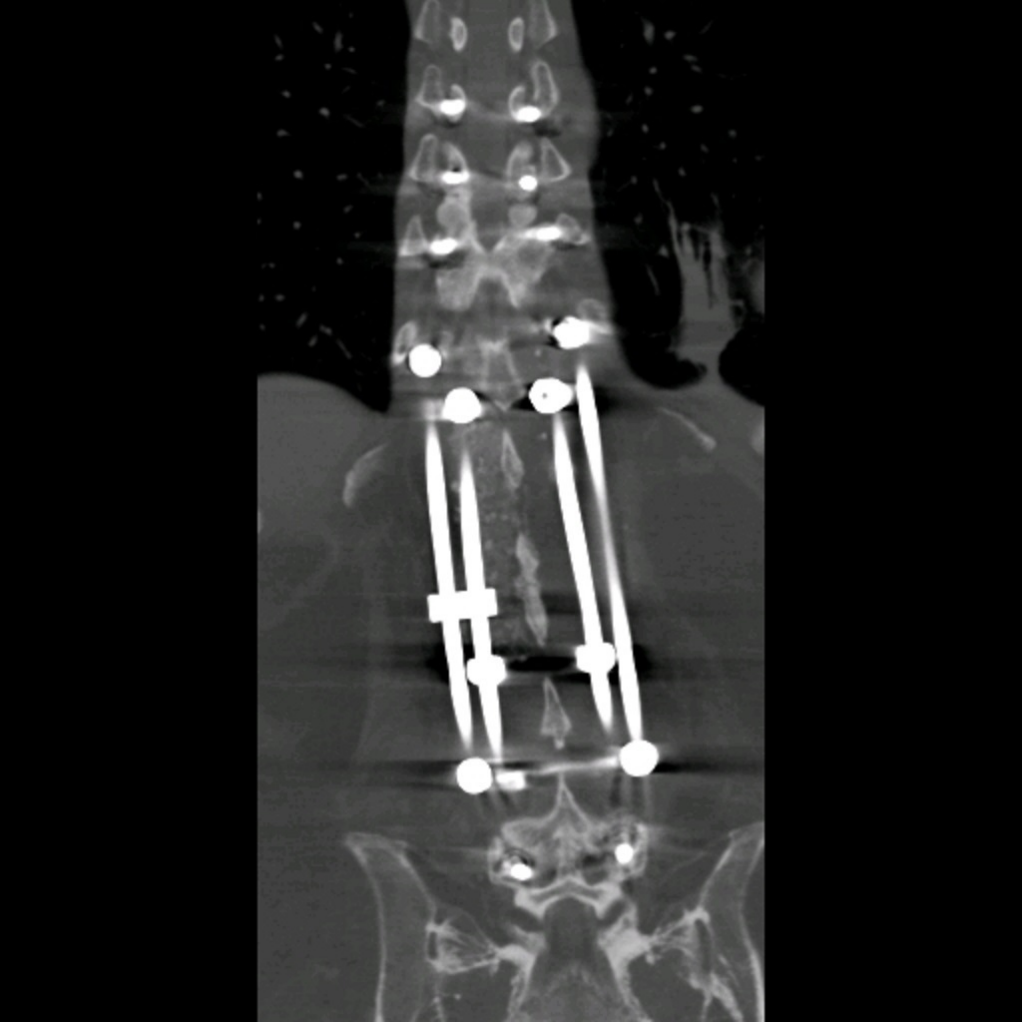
Coronal postoperative CT This coronal view of the postoperative CT of the thoracolumbar spine demonstrates the four-rod construct that was utilized. Medial and lateral entry points for pedicle screw placement were alternated from T8-12 and L3-5, as shown, prior to rod placement and utilization of rod-to-rod connectors.
